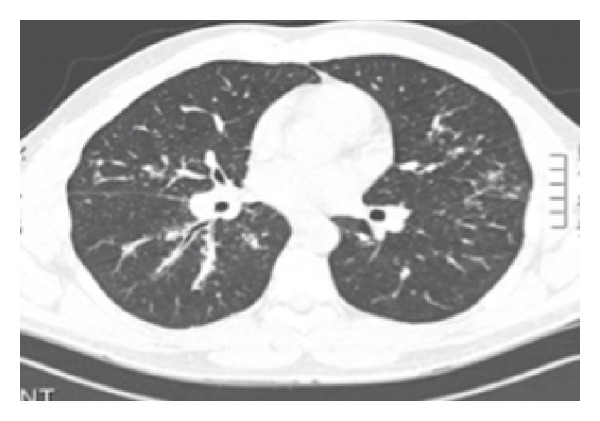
Pulmonary computed tomography showing bilateral hilar adenopathy and parenchymatous infiltration.
